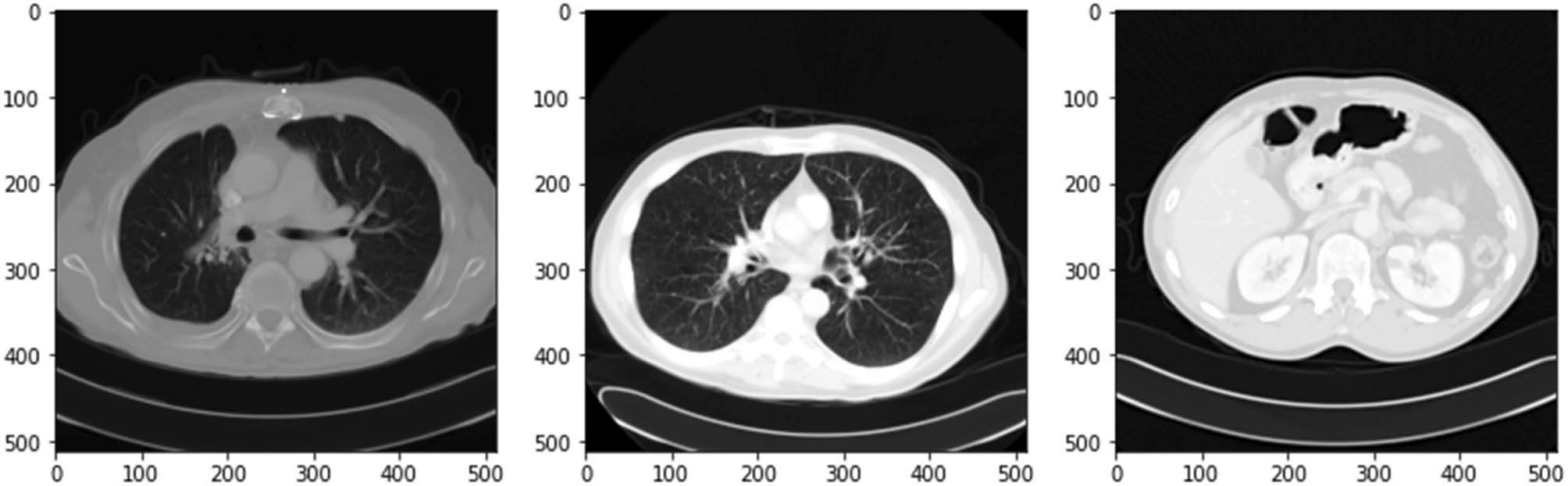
Sample CT scan images from the dataset.
